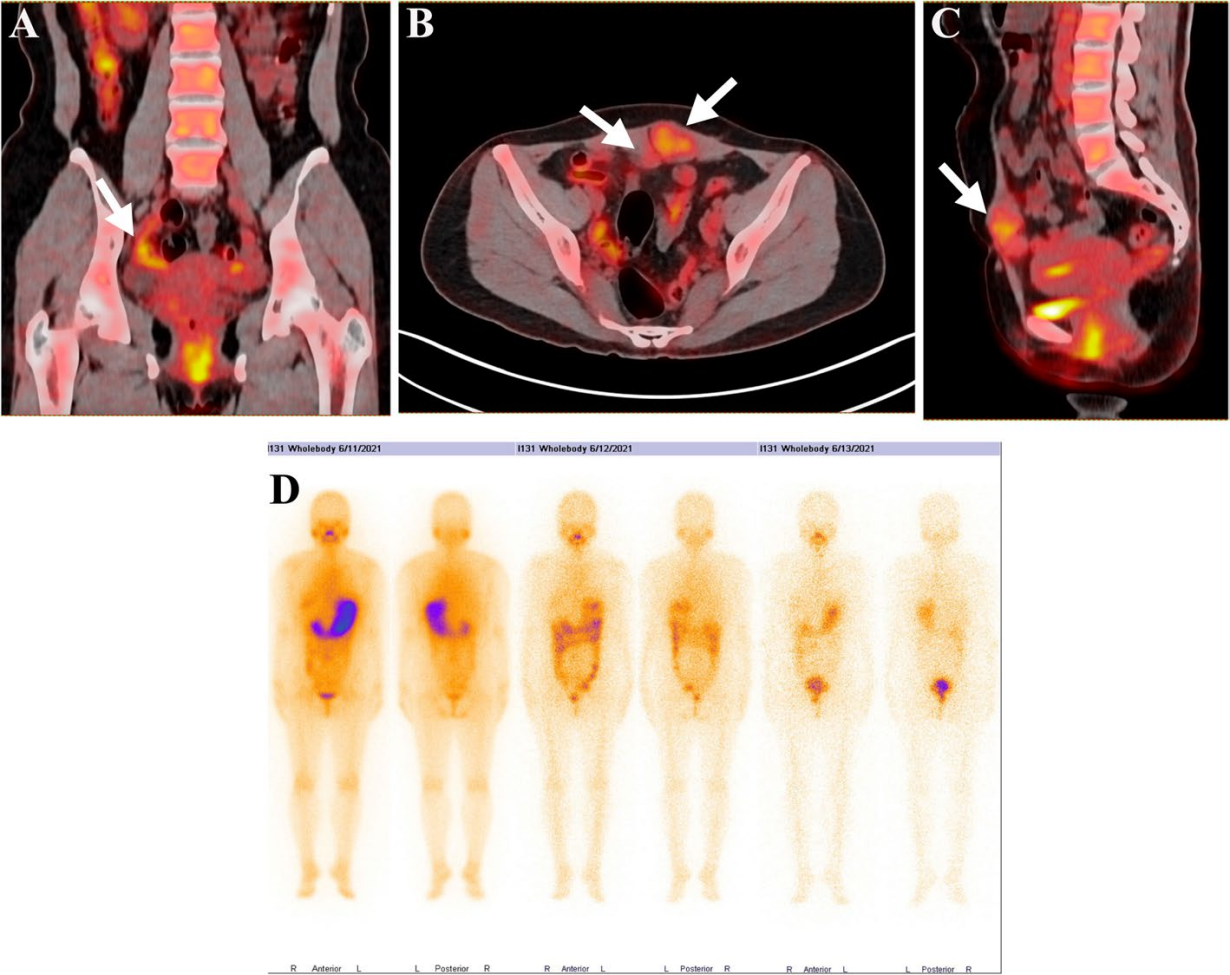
A, B, C PET-CT revealed the increased FDG uptake in abdominopelvic cavities. D No residual I.^131^uptake
